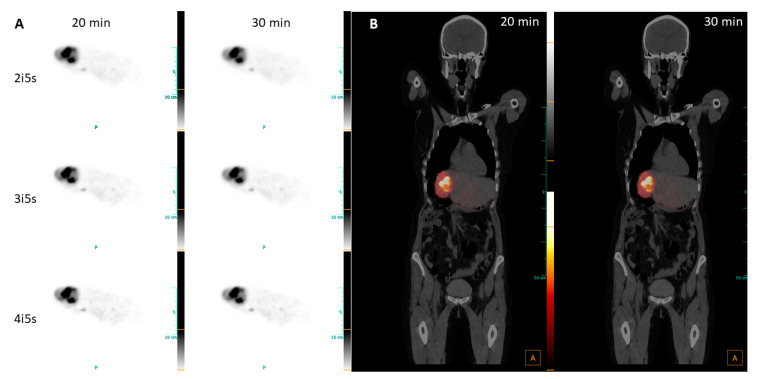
(A) Transversal views of PET images of patient 3, diagnosed with NET metastases, reconstructed with 2i5s, 4 mm filter; (B) fused coronal PET/CT images, reconstructed with 2i5s, 4 mm filter.
